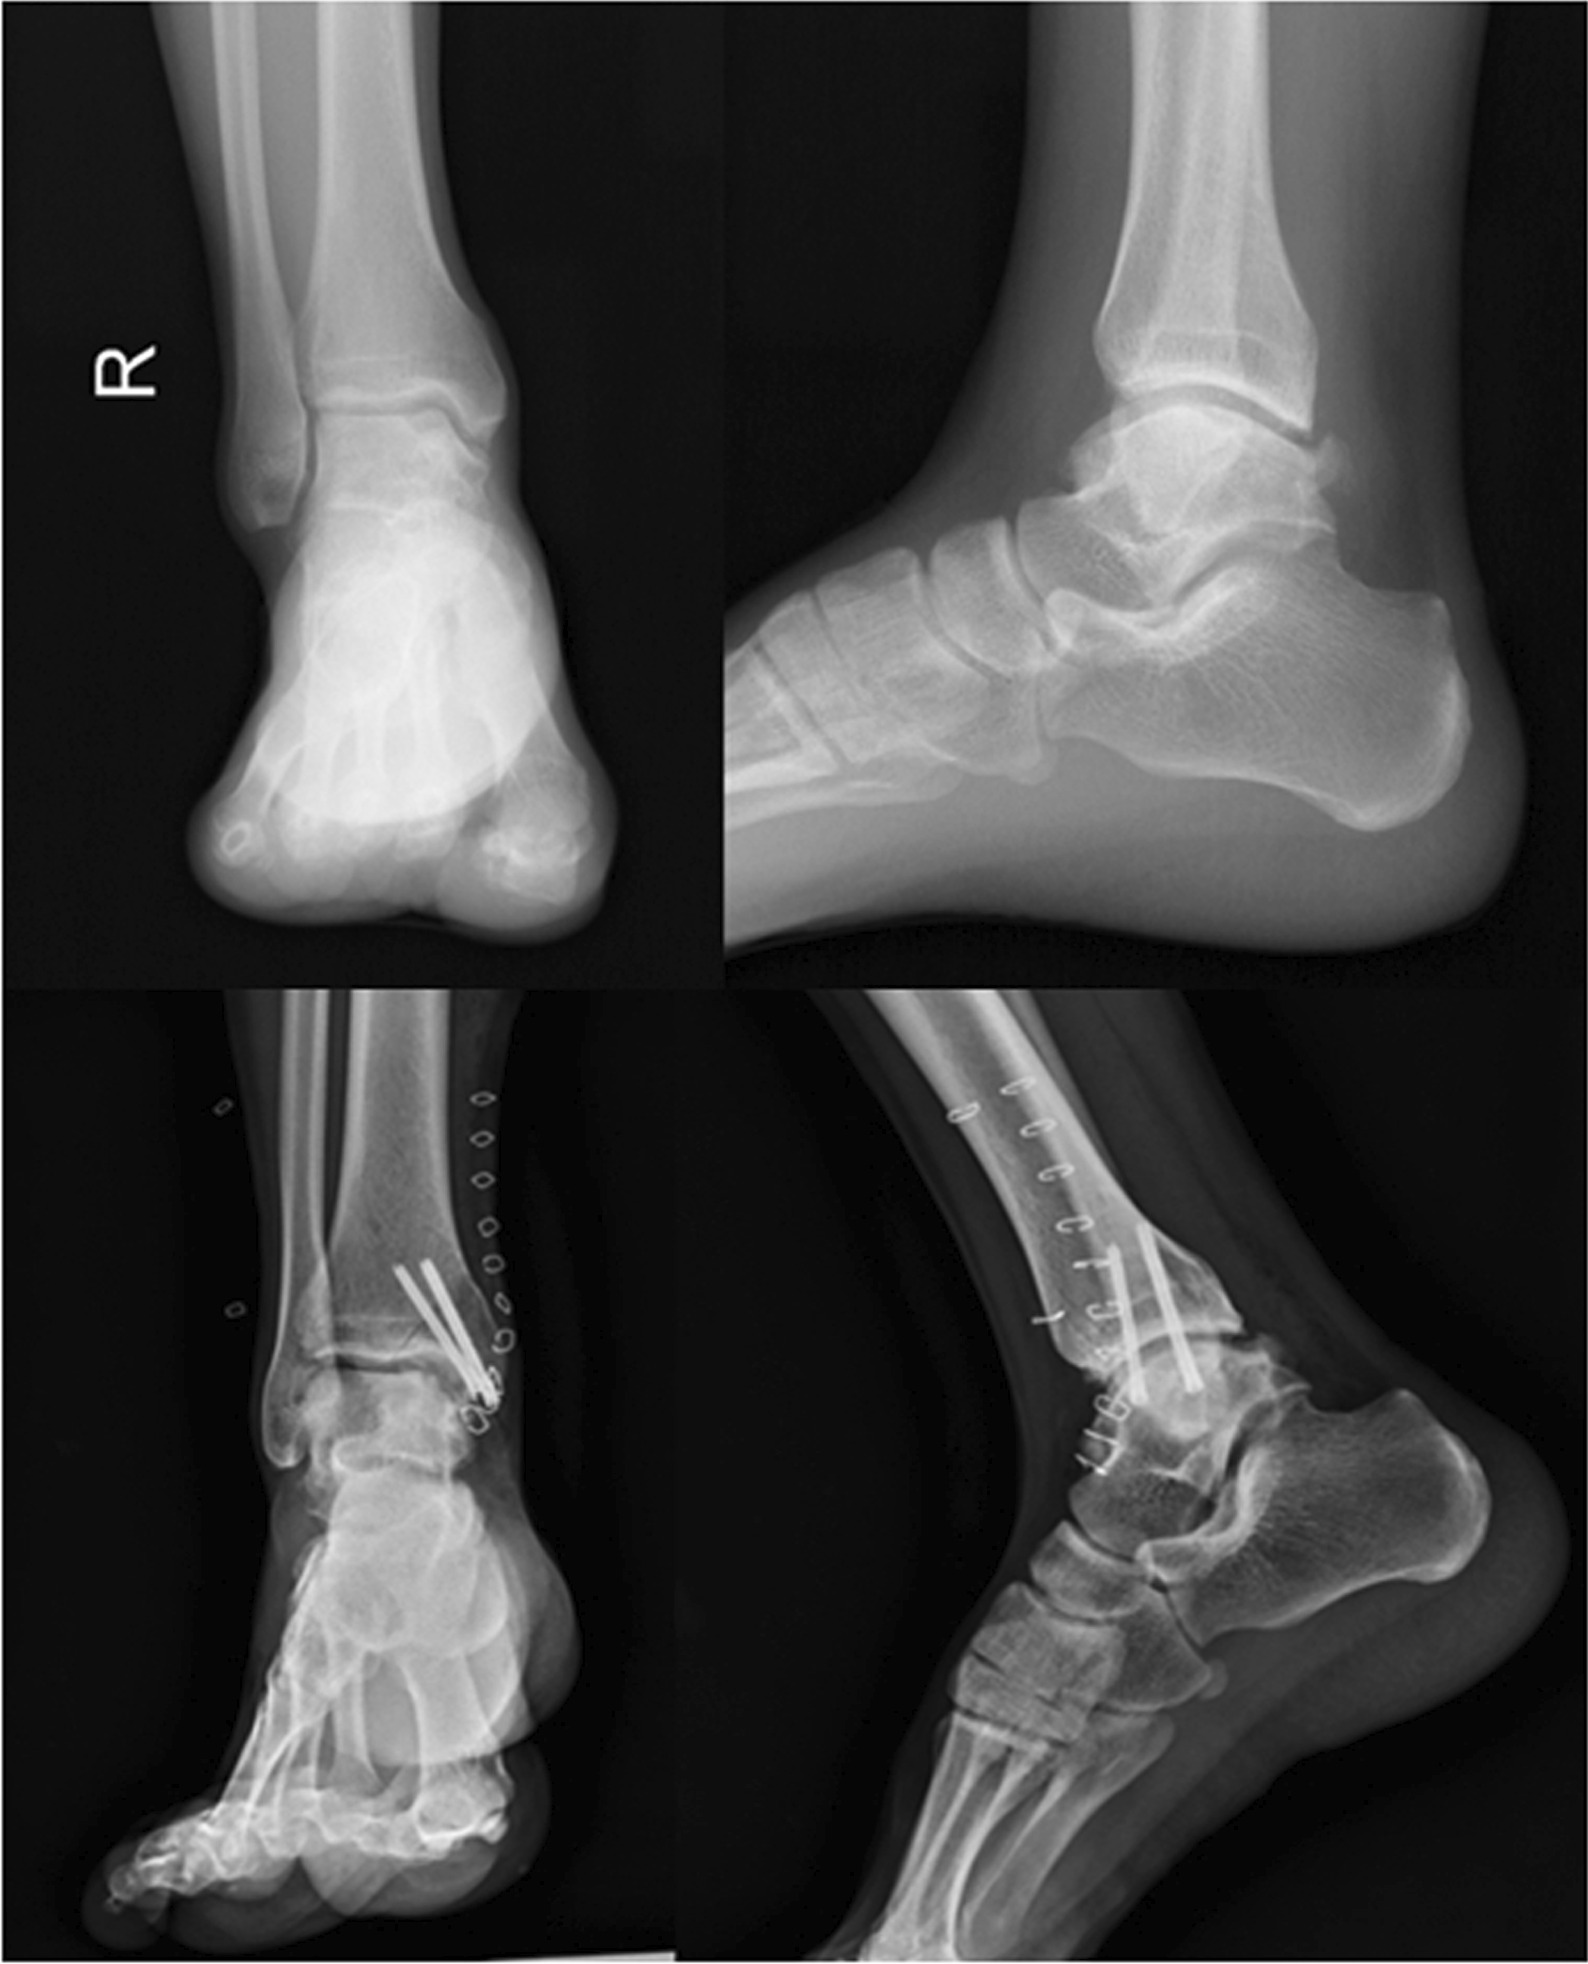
X-ray comparison before and after surgery
